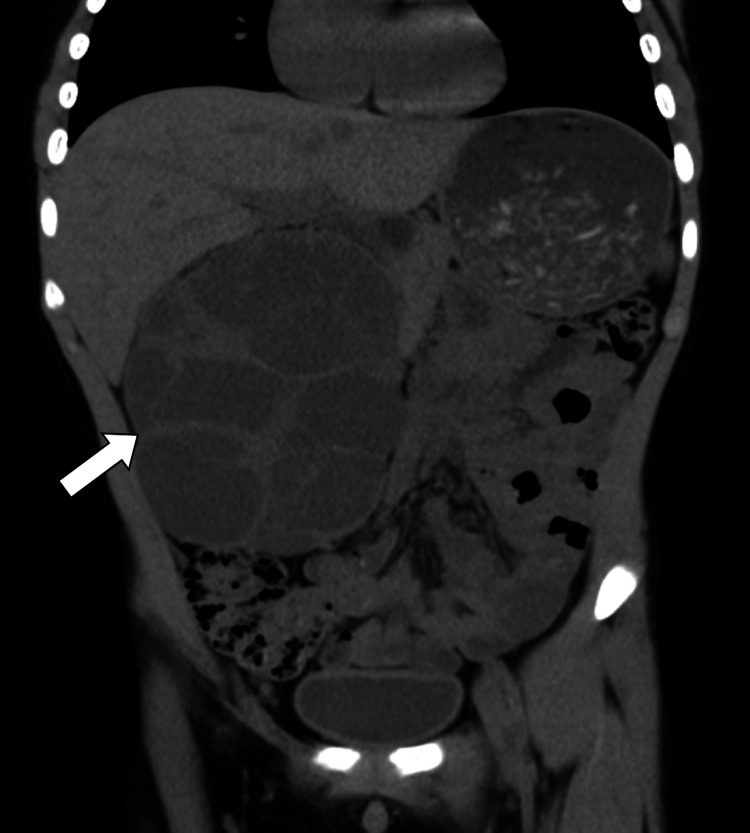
Coronal non-enhanced CT image showing clearly the multicystic nature of the large right kidney mass (arrow)
